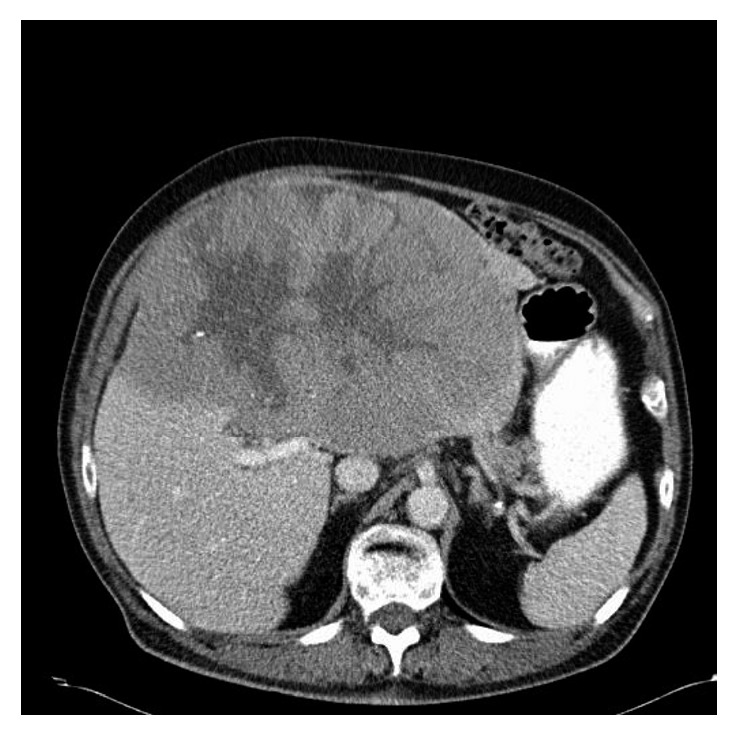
CT scan 24 months after the treatment.
